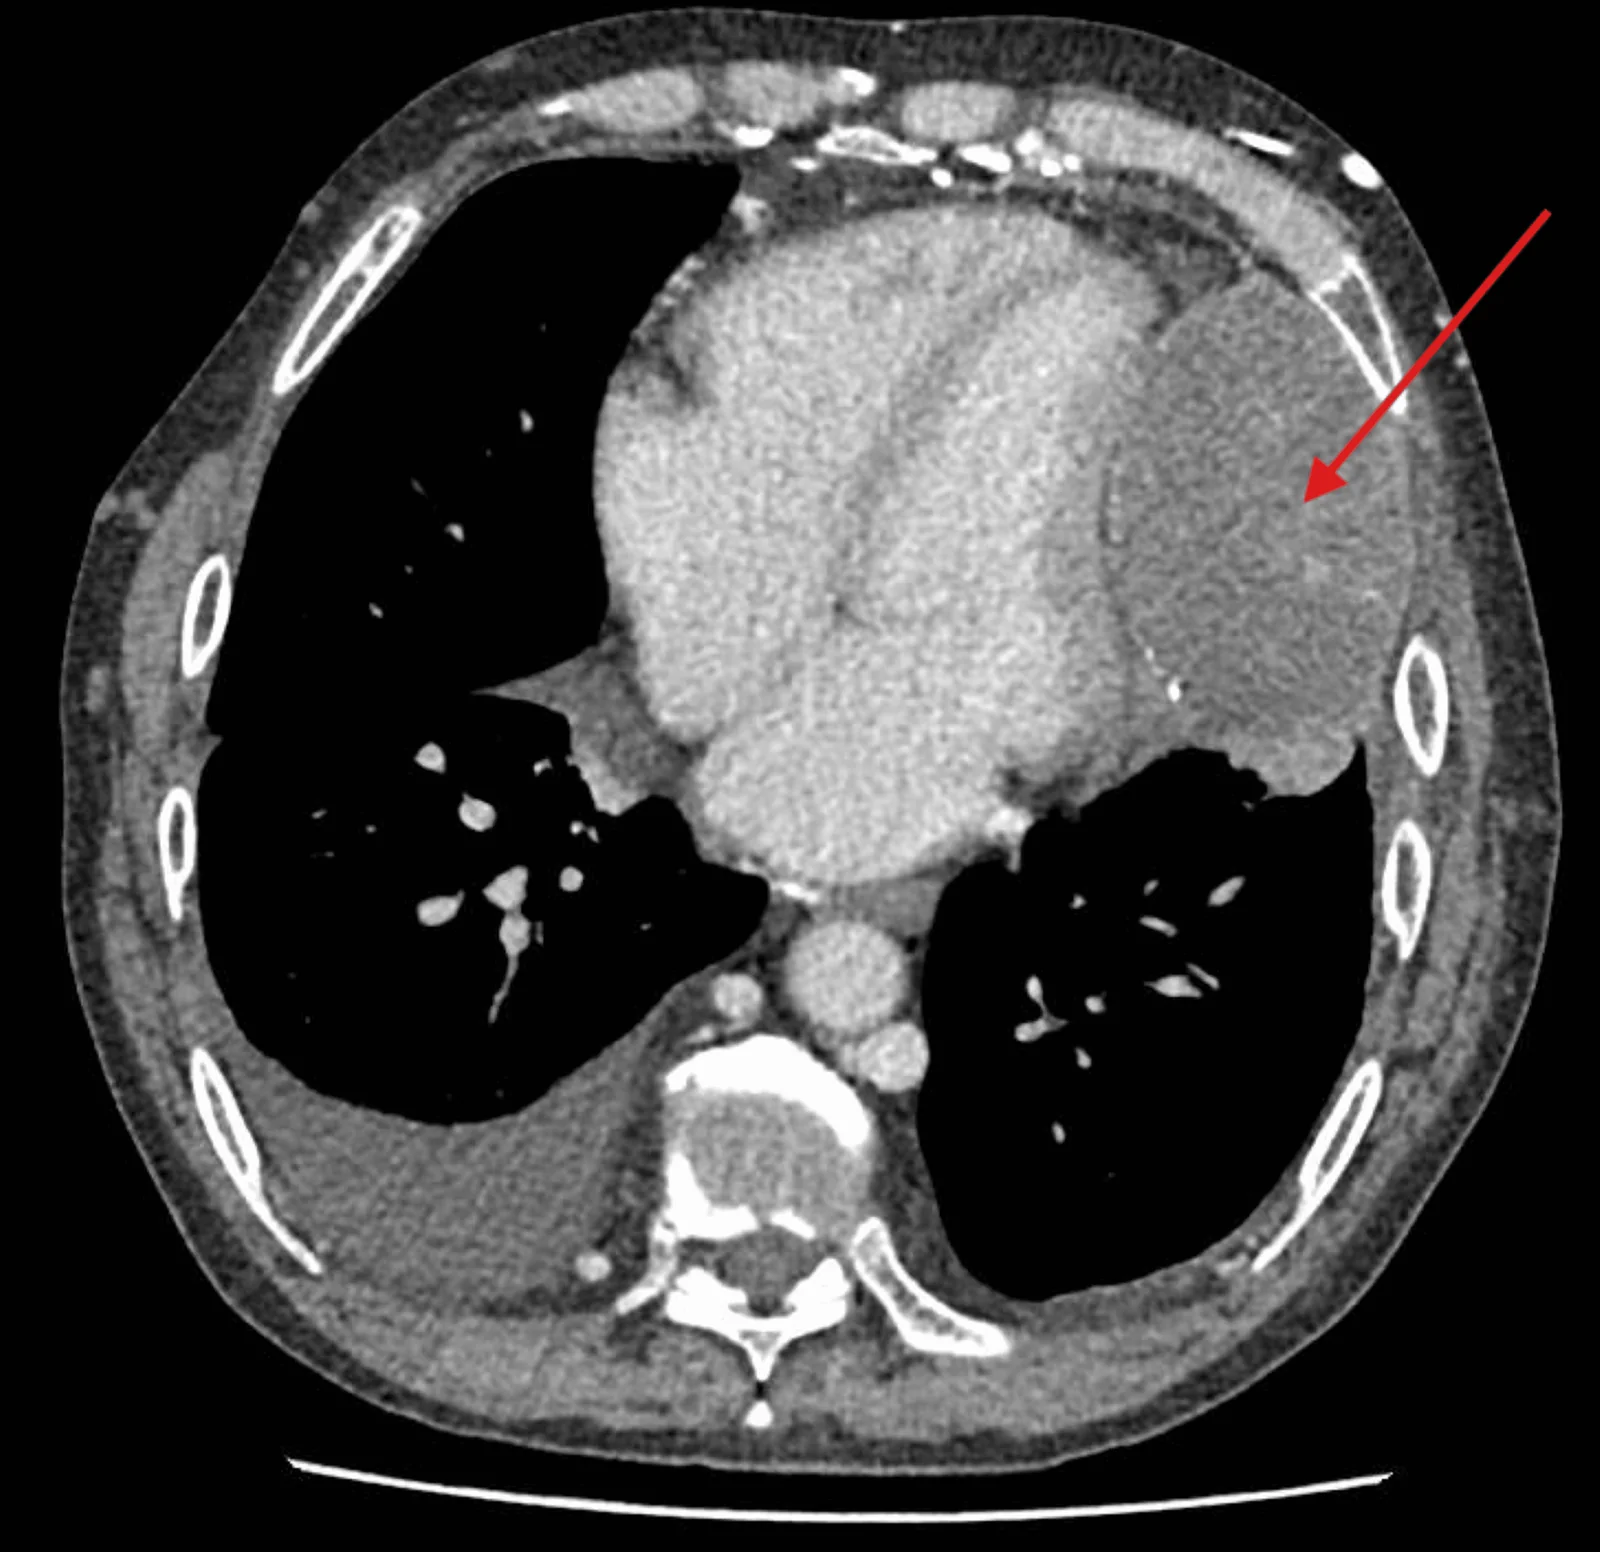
CT image showing the pleural haematoma (red arrow) causing compression on the left ventricle.
